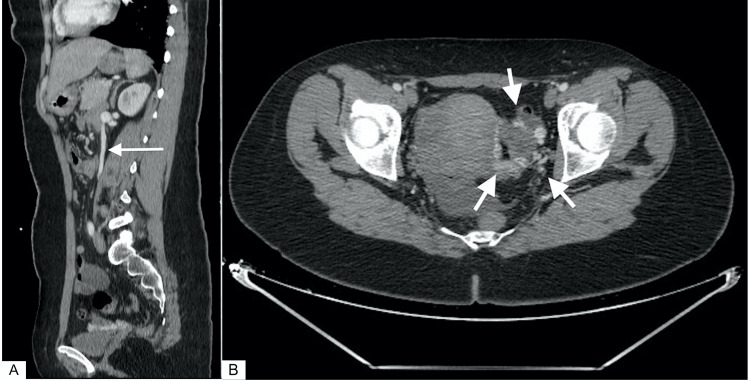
CT images of gonadal vein distension and pelvic congestion A: Dilatation of the left ovarian vein (white arrow) B: Pelvic venous circulation (white arrows)
